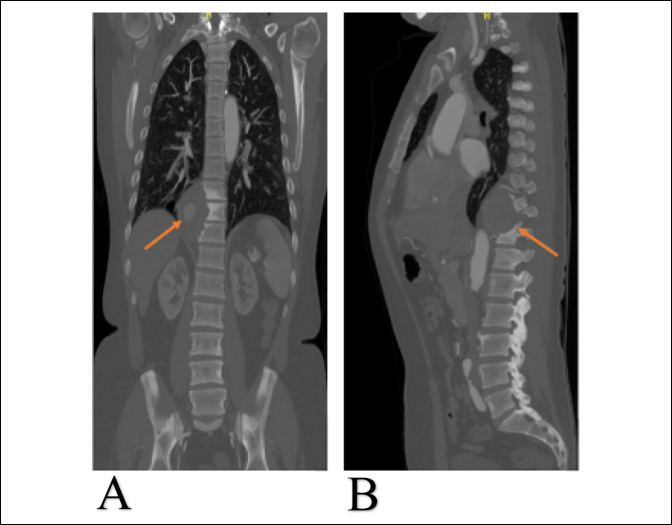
Radiograph showing the CT angiogram of the spine. A, Coronal view of pseudoaneurysm noted with arrows showing partial contrast enhancement inside the pseudoaneurysm. B, sagittal view of the pseudoaneurysm appearing as a mass spanning T-9 to T11.
